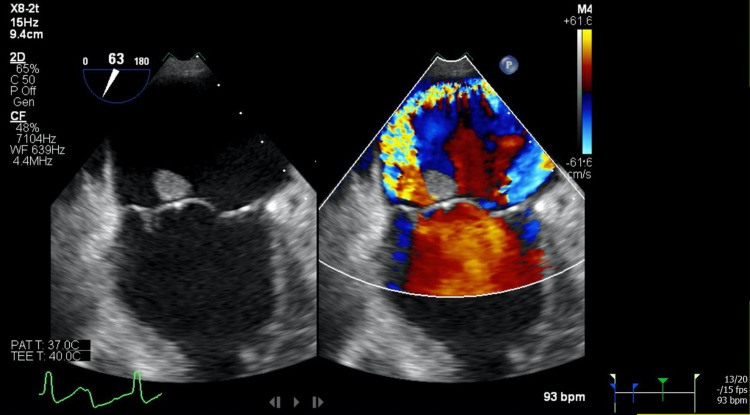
(Left) Transesophageal echocardiogram showing large mitral valve vegetation; (right) transesophageal echocardiogram with color doppler showing severe mitral regurgitation through the valve and perforated anterior leaflet
